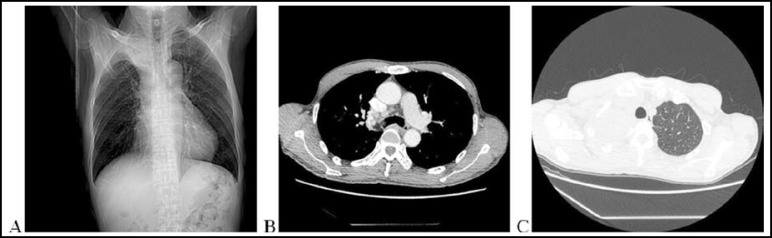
(A) Anterior-posterior chest X-ray showing atelectasis of the right upper lung. (B) Chest-computed tomography (CT) showing an extra-bronchial extension of tumor with low attenuation suggesting a fat containing lesion. (C) CT of the chest showing complete atelectasis of the right upper lobe secondary to bronchial obstruction (arrow).
